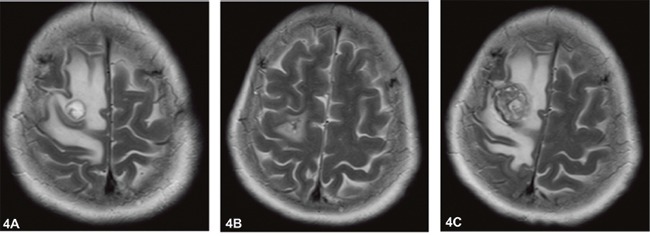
Magnetic resonance imaging of brain showed the mass located in the frontal lobe for Case 2 (A) Aug 27, 2014: at initial diagnosis; (B) Oct 16, 2014: after the first surgery; and (C) Feb 3, 2015 at the time of postoperative recurrence.
